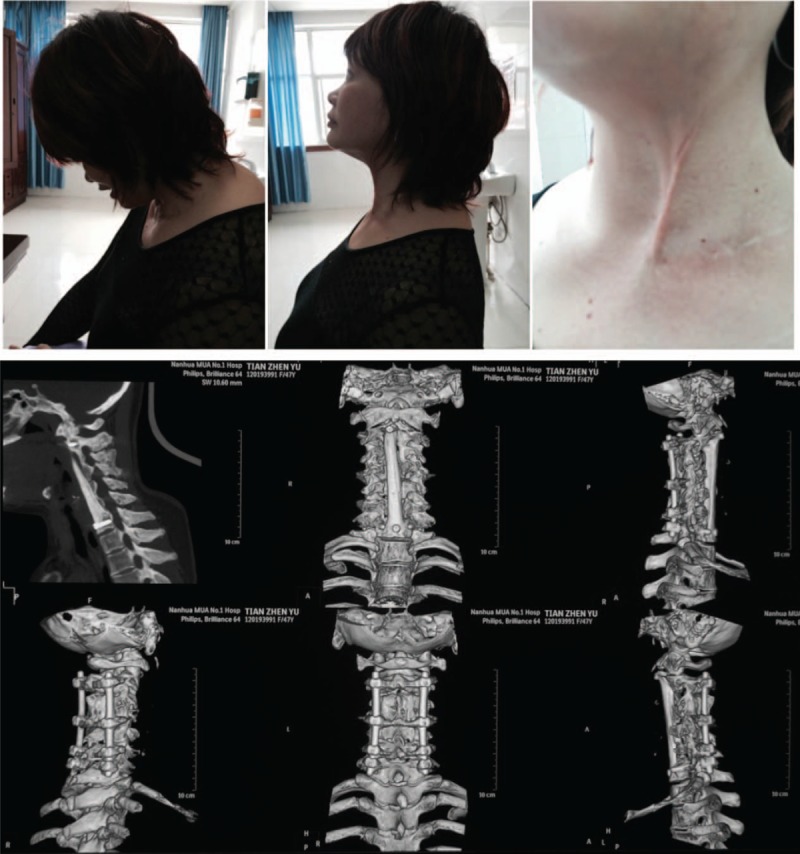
The radiographic image analysis at 28 months post-op shows strong internal fixation and good fusion. There were no signs of TB recurrence. The location of the internal fixation device was proper. Postoperative outcomes were measured using the patients’ cervical range of motion (ROM). TB = tuberculosis.
